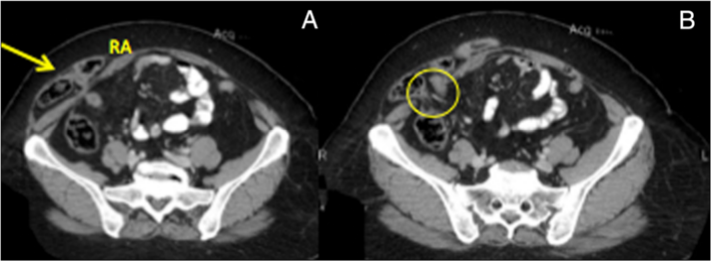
CT imaging of right Spigelian hernia. a) Hernia sac (arrow) containing loop of small bowel (RA: Right rectus abdominis); b) Abdominal wall defect (O)
